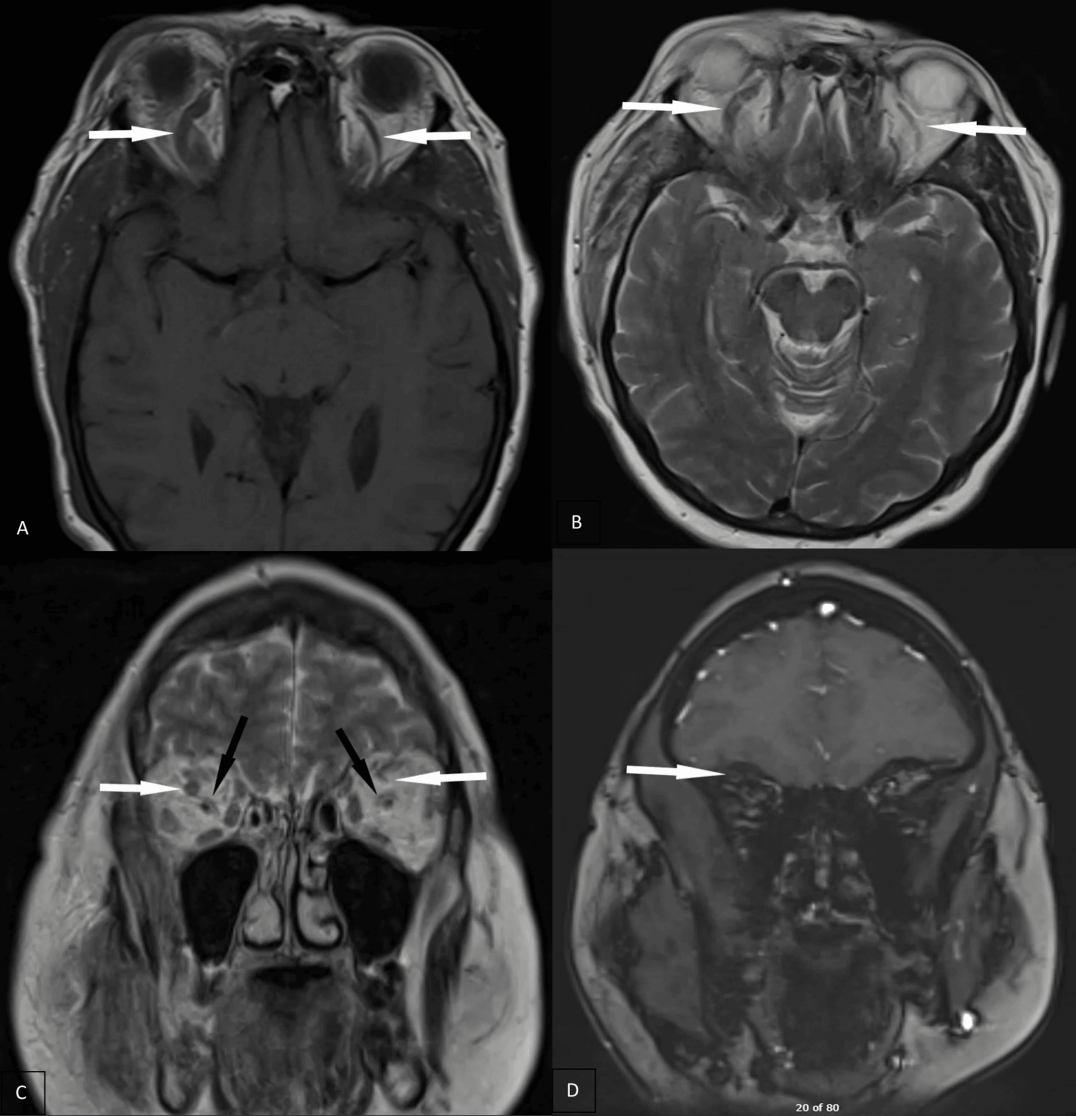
MRI images of the brain showing findings of right superior ophthalmic vein thrombosis. The axial T1-weighted image and axial T2-weighted image demonstrate asymmetric enlargement of the right superior ophthalmic vein (white arrows, A and B). The coronal T2-weighted image shows enlargement of the right superior ophthalmic vein demonstrated in another plane (white arrows, C) with normal appearance of the optic nerve and sheath bilaterally (black arrows, (C). The coronal T1-weighted post-gadolinium image shows a faint intraluminal filling defect within the right superior ophthalmic vein compatible with right superior ophthalmic vein thrombosis (white arrow, D).
